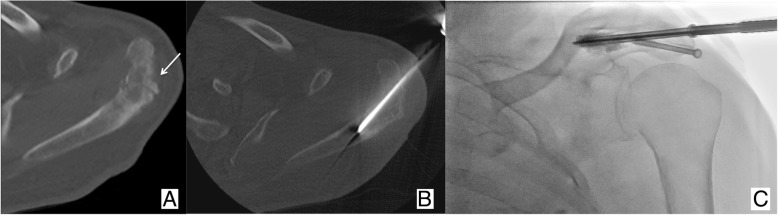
Seventy-four-year-old male patient affected by lung cancer. The patient underwent radiation therapy of a painful metastasis of the left acromion, which was complicated few weeks later by (a) a secondary bone insufficiency fracture. b, c Percutaneous osteosynthesis was proposed to fix the fracture under combined CT and fluoroscopy guidance; two screws were deployed with subsequent rapid pain relief
